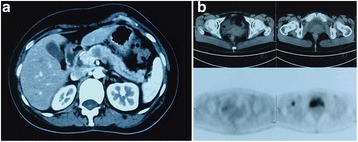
a: Contrast-enhanced computed tomography scan of the abdomen showing a low-density mass confined to the neck of pancreas. b: Positron emission tomography/computed tomography scan of the pelvis: a lytic bony lesion in the right caput femoris, with abnormal uptake of fluorodeoxyglucose
